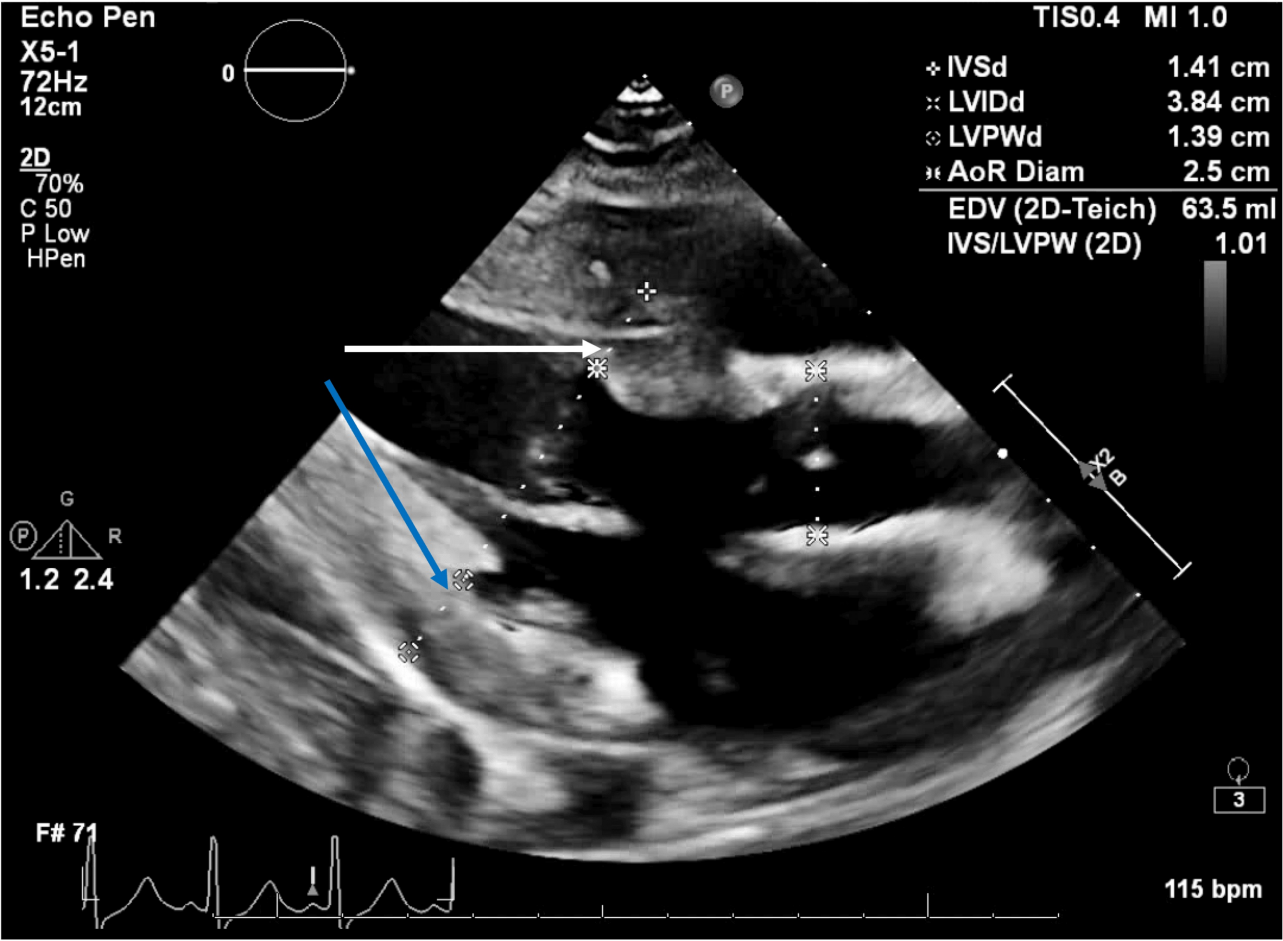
Echocardiogram indicating left ventricular hypertrophy The patient’s mildly elevated IVSd (1.41 cm; normal ≤ 1.0 cm, white arrow) and LVPWd (1.38 cm; normal ≤ 1.0 cm, blue arrow) indicate mild left ventricular hypertrophy. Measurements are indicated by calipers and corresponding values in the upper right corner. Abbreviations: IVSd: Interventricular Septal Thickness in Diastole; LVPWd: Left Ventricular Posterior Wall Thickness in Diastole
